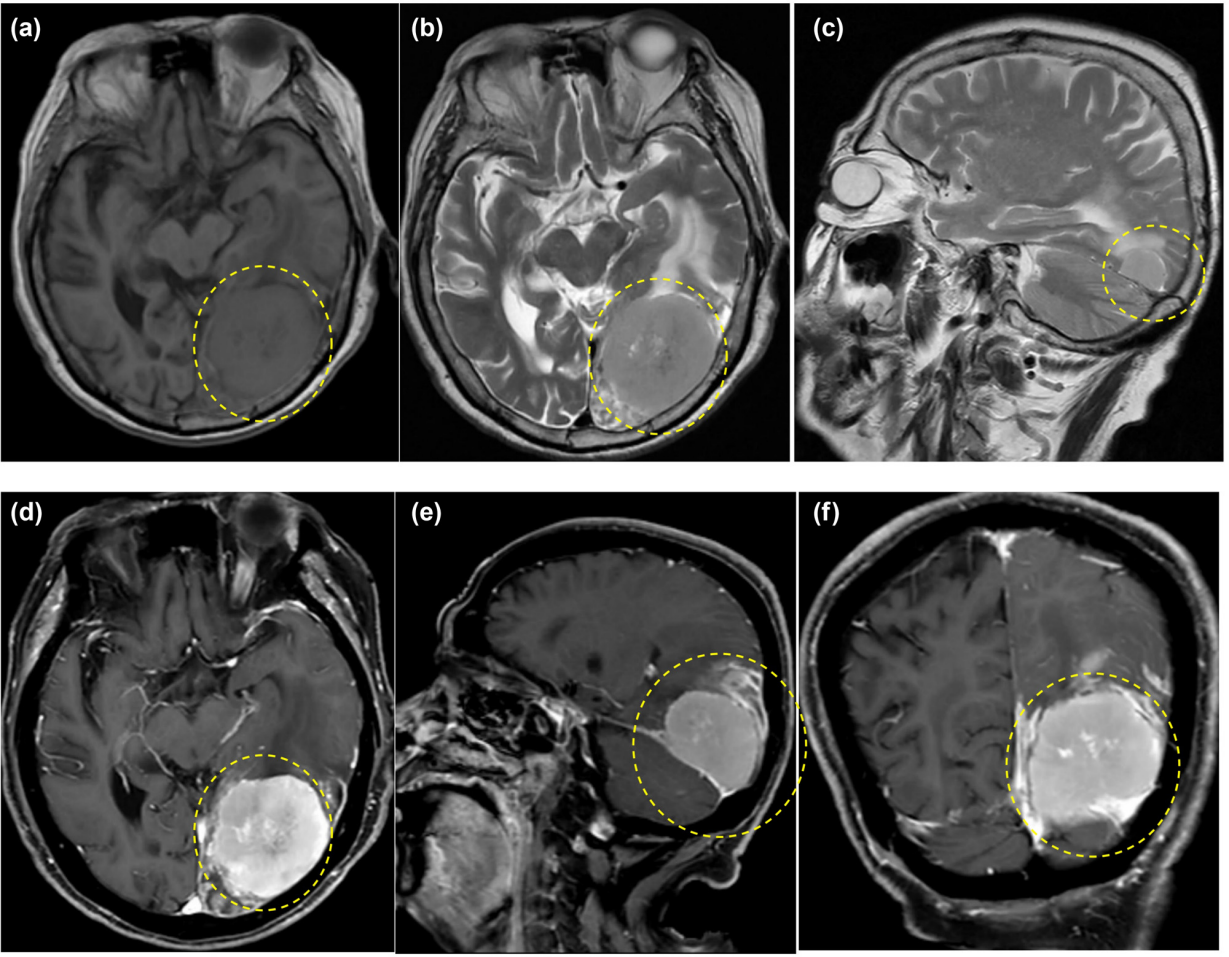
Brain MRI images demonstrating a large left-sided parasagittal occipital extra-axial mass with heterogeneous enhancement, with the base of the tumor located on the tentorium cerebelli (yellow dashed circle). The mass is isointense to grey matter on T1-weighted images and hyperintense on T2-weighted images (a)–(c); the calcified components are hypointense on T2-weighted images. There is marked contrast enhancement on T1-weighted images with gadolinium (d)–(f).
